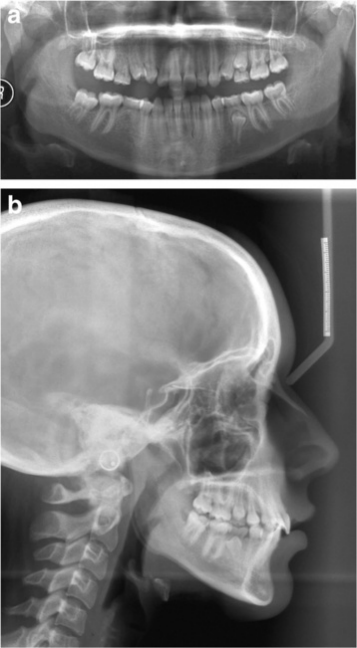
a Panoramic radiograph showing congenitally missing right 2nd premolars. b Cephalometric radiograph at the start of treatment
